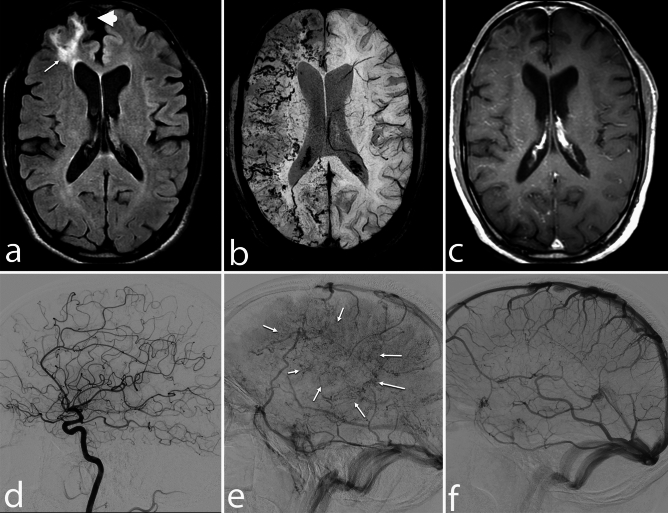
Atypical unilateral PCNSV. A 39-year-old man presented with chronic headaches and sudden onset left hemiparesis. In MRI, axial FLAIR image (a) shows a well-defined area of hyperintensity in the subcortical and periventricular white matter of the right anterior frontal lobe (arrow) with dilation of adjacent cortical sulci (arrowhead) and iplitaeral frontal horn. Axial SWI image (b) shows multiple curvilinear and punctate foci of blooming in the right cerebral hemisphere. Note that the extent of blooming on SWI is quite extensive compared to FLAIR signal abnormality. Post-contrast T1-WI (c) shows a perivascular and punctate pattern of enhancement in the right cerebral hemisphere. Cerebral DSA images (d, e) of the right cerebral hemisphere show a pseudophlebitis pattern in delayed venous phase (e) with no obvious abnormality in the arterial phase (d). DSA image (f) shows a normal venous angiogram of the left cerebral hemisphere. These findings are compatible with the unilateral pattern of PCNSV.
